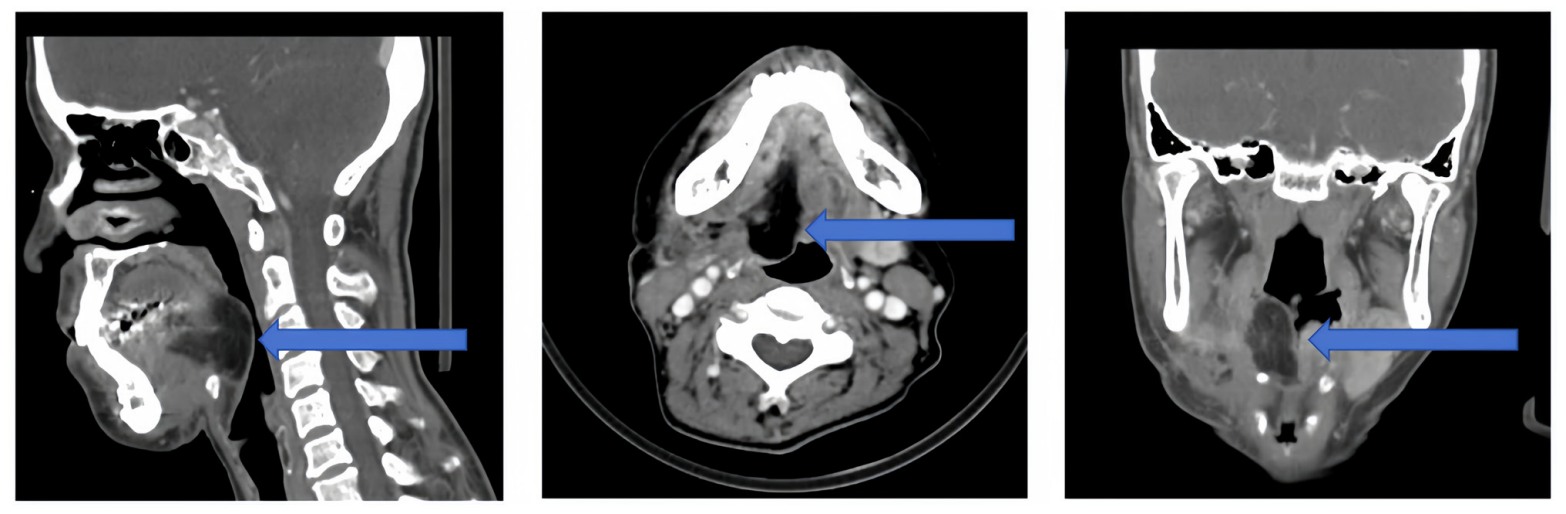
Postoperative enhanced computed tomography of the tongue. The image revealed discontinuity in the alignment of the cortical and mandibular bones. The right side of the tongue was partially missing, and the structure of the operative area was disorganized (arrow). The right submandibular gland was not visible, and the operative area appeared as a dense nodular shadow. Pore patches of adipose tissue accumulation and soft tissue density shadows were observed from the operative area to the right sternocleidomastoid muscle. The enhancement scan of the operative area showed linear enhancement of density shadows. The right submandibular area of soft tissue was slightly swollen, and the surrounding fat interstitial space was slightly turbid. The right laryngopharyngeal and oropharyngeal cavities were slightly narrowed, indicative of postoperative change.
